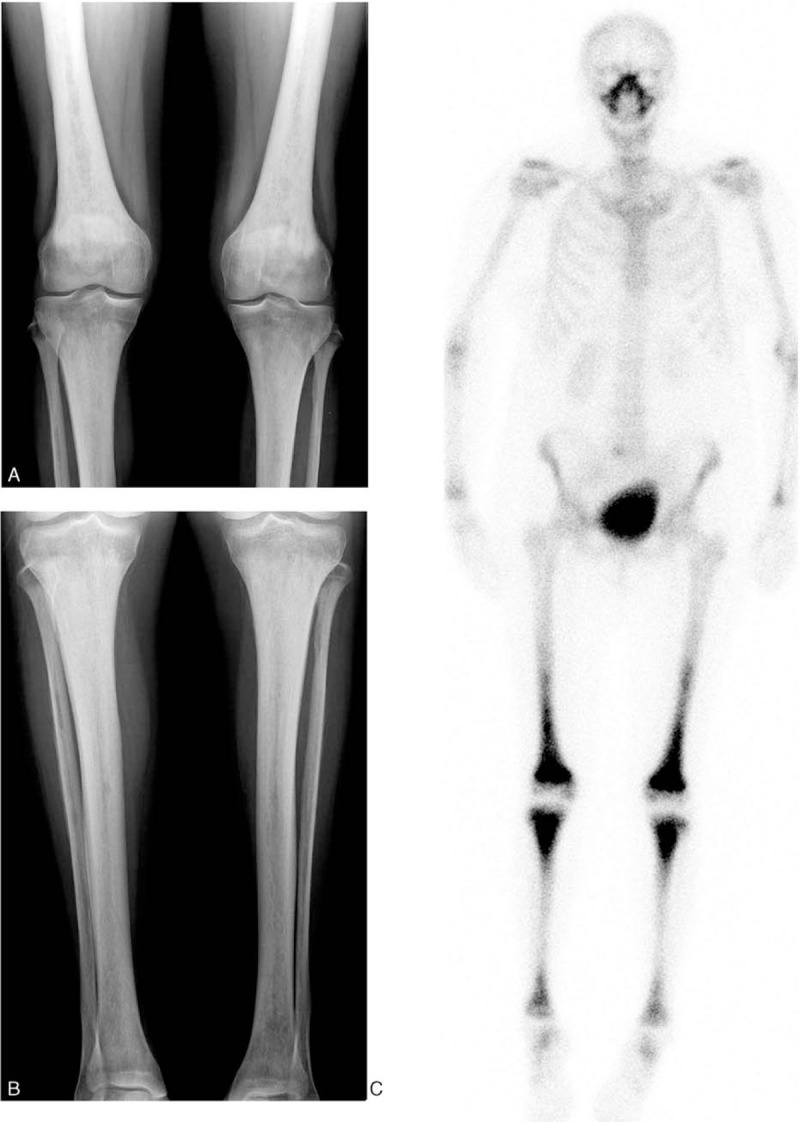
X-rays (A, B) shows bilateral and symmetric cortical osteosclerosis of the diaphyseal and metaphyseal regions in the long bones of the lower limbs with symmetrical hyperfixation on ^99m^Technetium bone scintigraphy (C).
